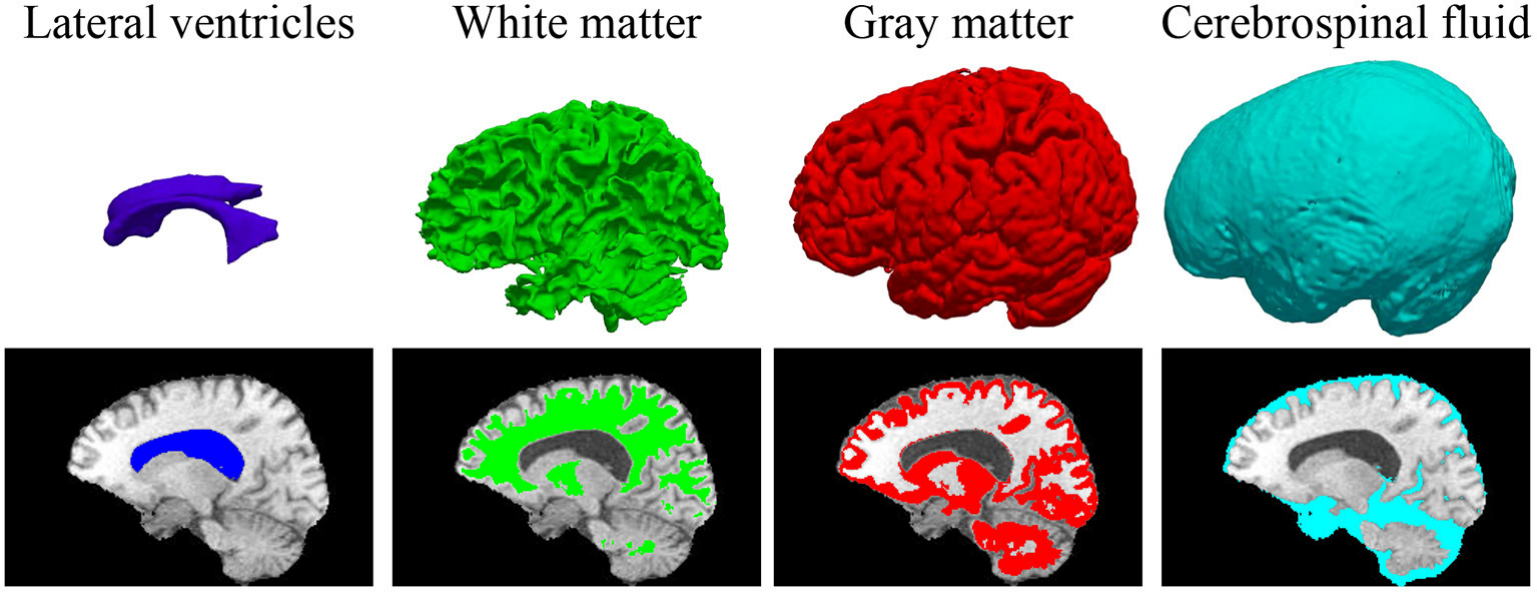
3D displays and overlayed T1 MR images of the four brain parcellations for a representative case from the BraTS dataset.
